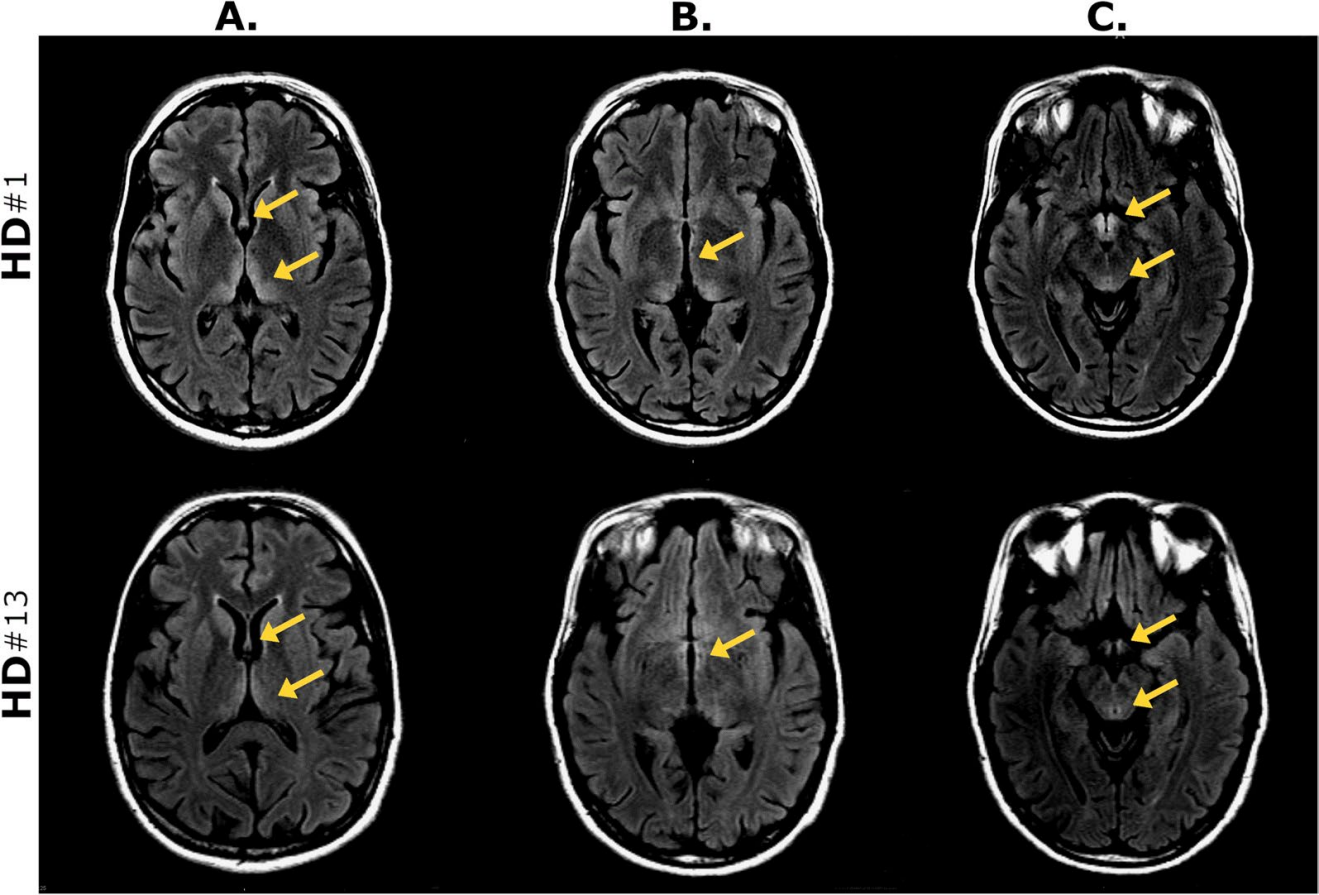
Magnetic resonance imaging (MRI) of the head using T2-weighted Fluid-Attenuated Inversion Recovery (T2/FLAIR) sequences obtained on hospital day 1 and repeated on transfer on HD13 demonstrates hyperintensities in the A fornix, bilateral medial thalami, B third ventricle, and C mammillary bodies and periaqueductal gray that leads to the floor of the fourth ventricle (not pictured). Aforementioned findings are demonstrated by the yellow arrows. The diagnosis of Wernicke Encephalopathy can be confirmed with these MRI scans demonstrating abnormalities; however, it is important to note that it is not sensitive as negative findings do not exclude the diagnosis of Wernicke–Korsakoff syndrome
